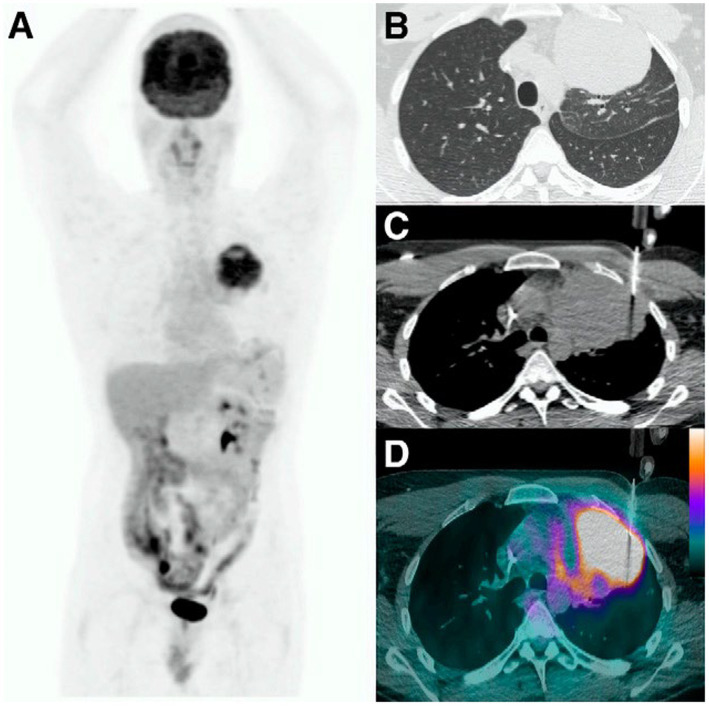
PET-CT fusion image confirms that the puncture reaches the high metabolic. (A) Maximum-intensity-projection PET image. (B) Transaxial CT lung window. (C) Transaxial CT mediastinal window (intraprocedure). (D) Transaxial ^18^F-FDG PET/CT image (intraprocedure CT image fused with previously acquired PET/CT image).
